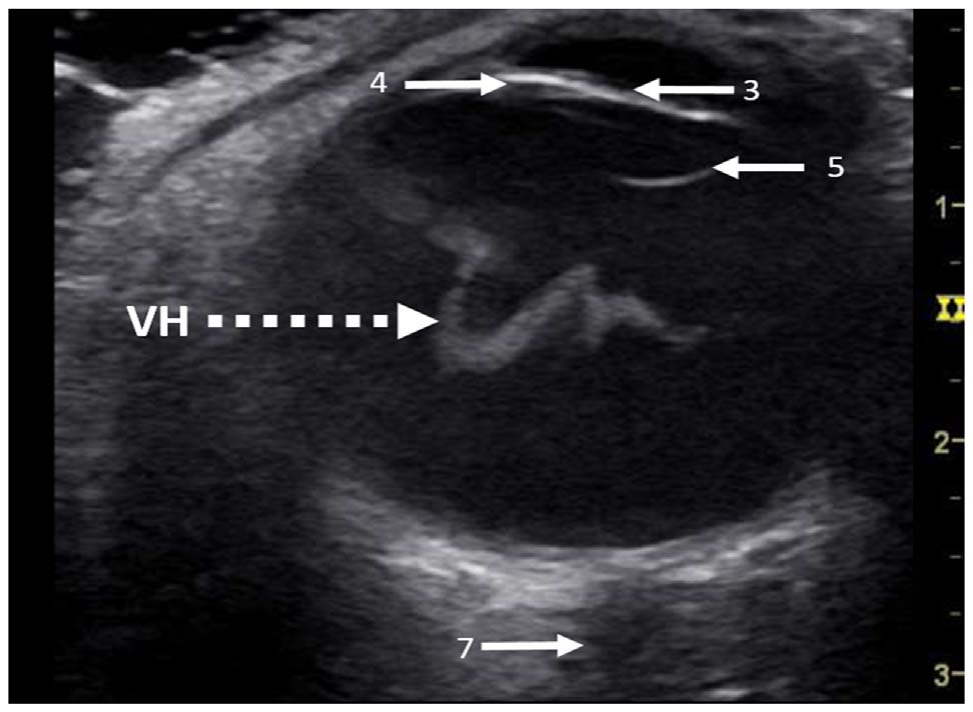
Ocular ultrasound examination of a vitreous haemorrhage (VH) in the left eye. In this case Terson's syndrome appeared as a hyperechogenic membrane caused by clotted blood within the vitreous body. According to Fig. 1 iris and ciliary bodies (4) as well as the anterior (3) and posterior reflection (5) of the lens and the optic nerve (7) are visible.
